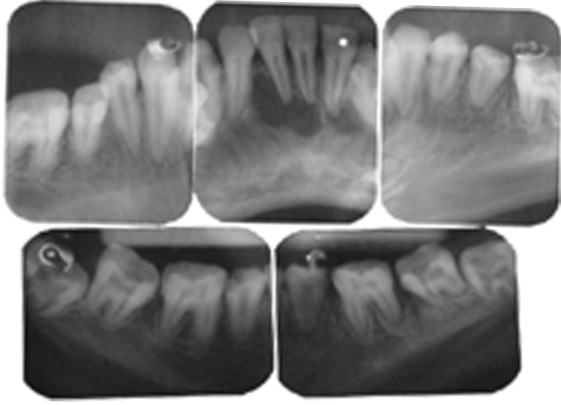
IOPARs of the dentition in the lower arch showing periapical radiolucency in relation to lower right and left central and lateral incisors
